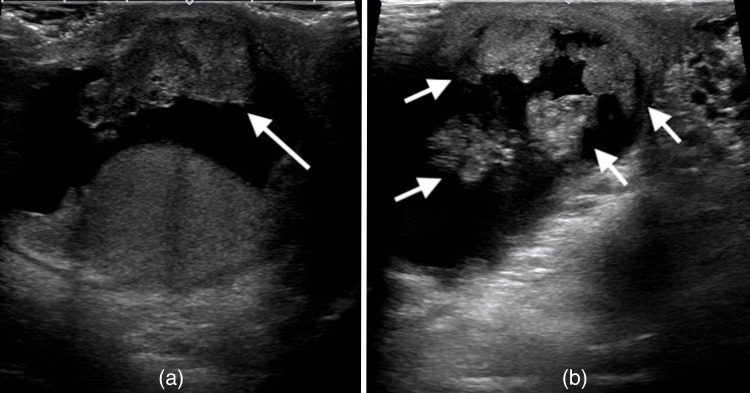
Ultrasonography of the abdomen. (a) A 33 mm mass (long arrow) in the right inguinal region. (b) Several nodules (short arrows) in the right inguinal region.
